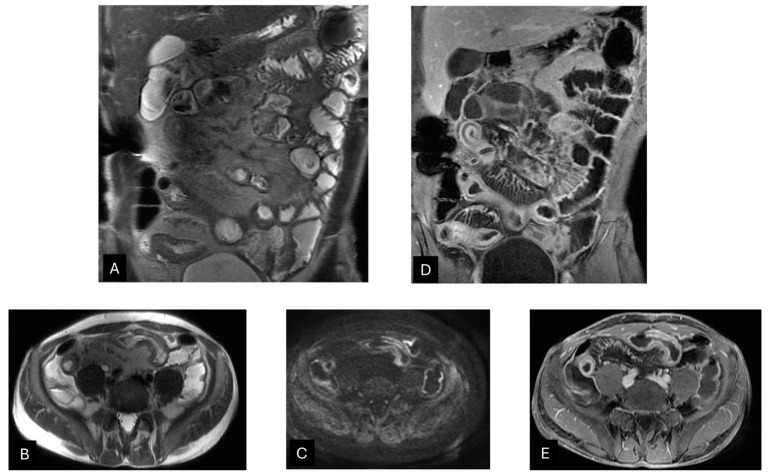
A 56-year-old man suffering from Crohn’s disease affecting the mid-distal section of the ileum with stenotic/substenotic and ecstatic features. Coronal (A) and axial (B) T2WI SSFSE images show multiple wall thickenings with a high T2 signal at the level of the ilium. The axial DWI (*b* = 1000) (C) image reveals increased intramural signal intensity, indicating restricted diffusion of the multiple thickened tracts. Coronal (D) and axial (E) post-contrast T1WI demonstrate intense wall structure multilayer enhancement.
